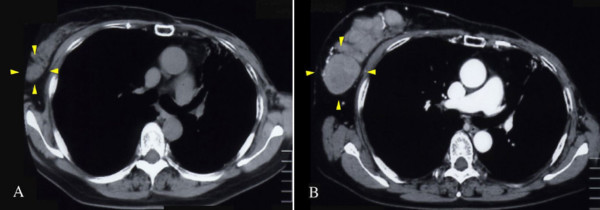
Chest CT images. A) CT was performed on November 22, 2005. B) CT was performed on December 21, 2005. The tumour apparently enlarged during a month. The doubling time was calculated at the same area (arrow) of the tumour.
